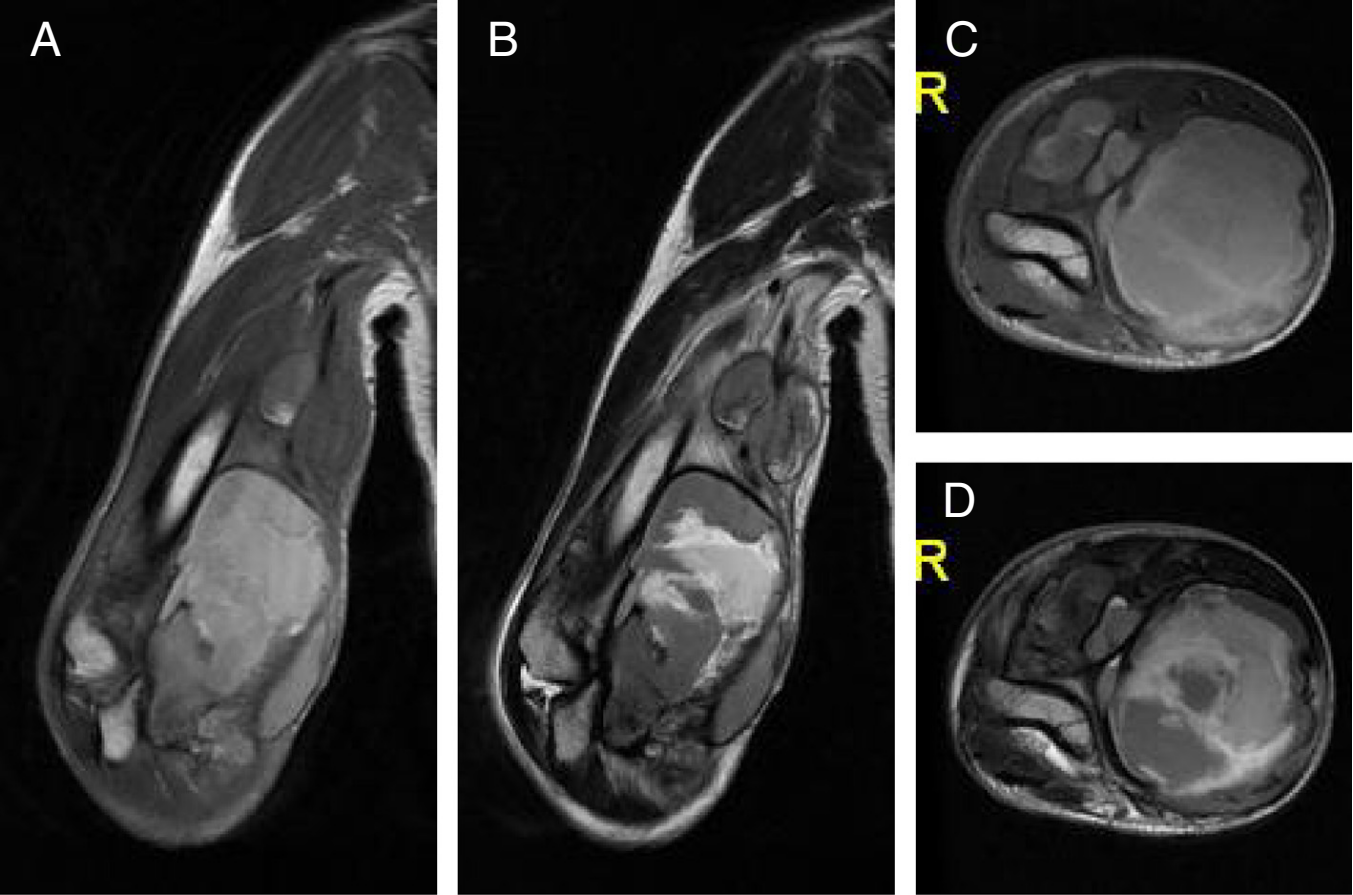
MRI of the huge tumor in the right upper extremity of a 67-year-old man. (A) T1-weighted coronal image. (B) T2-weighted coronal image. (C) T1-weighted axial image. (D) T2-weighted axial image.
